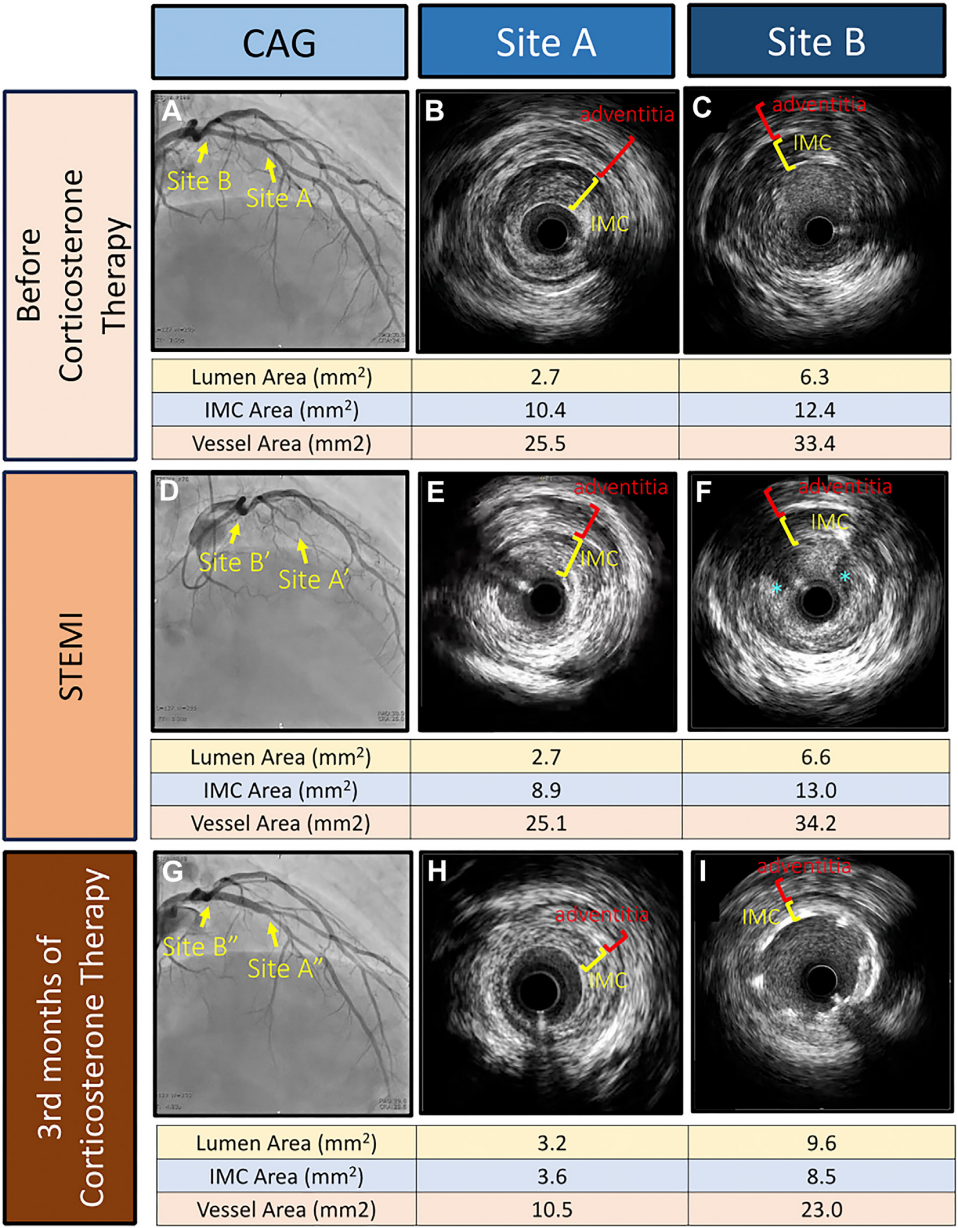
CAG and Intravascular Ultrasonography of the Left Anterior Descending Coronary Artery The most stenotic site (site A) and the culprit site in acute coronary syndrome (site B) are shown. (A) Coronary angiography (CAG) showed stenosis of the left anterior descending coronary artery. (B and C) Intravascular ultrasonography showed adventitia thickening with obvious vessel area enlargement. Intravascular ultrasonography also detected intima-media complex (IMC) thickening with nonhomogeneous low echogenicity. (D) Coronary angiography in ST-segment elevation myocardial infarction on the fifth day of steroid therapy. (E) Intravascular ultrasonography at site A. (F) Intravascular ultrasonography image at the culprit site showed intramural thrombus (asterisks). (G and I) Coronary angiography and intravascular ultrasonography on the third month of steroid therapy showed no in-stent restenosis. (H) Intravascular ultrasonography showed the decrease in the adventitia and intima-media complex thickening.
